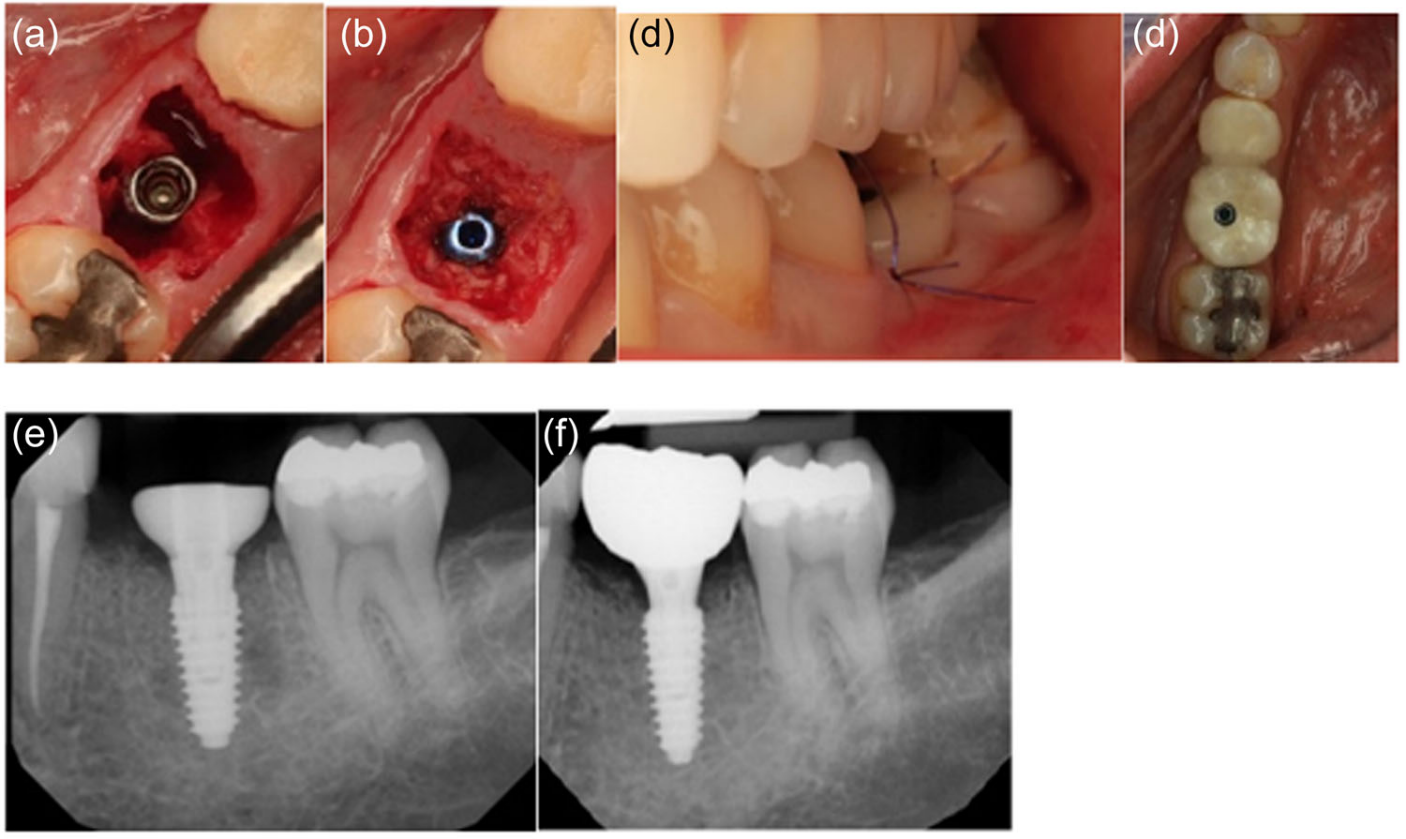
Minimally invasive removal of carious molar and placement of immediate implant without osteotomy on Patient 7. (a) Flapless and prepless Implant placement in molar socket. (b) Cover screw placed to accommodate grafting with cortical cancellous allograft. (c) Anatomical healing abutment placed to cover the entire socket and accommodate volume preservation. Sutures placed to re‐approximate papillae. (d) Final screw‐retained crown. (e) Peri‐apical radiograph on the day of placement. (f) Peri‐apical radiograph with final restoration at 12 months.
